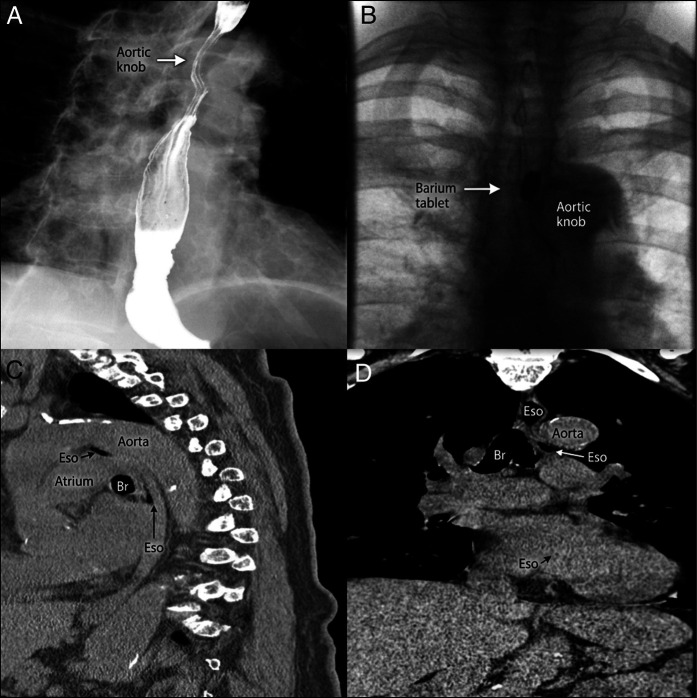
Dysphagia aortica on barium esophagram and CT. (A) Prominent aortic impression on the mid-thoracic esophagus. (B) 12.5 mm tablet transiently retained at the impression (arrow). (C) Sagittal CT showing esophageal indentation by the thoracic aorta. (D) Axial CT demonstrating focal esophageal narrowing adjacent to the aorta. CT, computed tomography.
